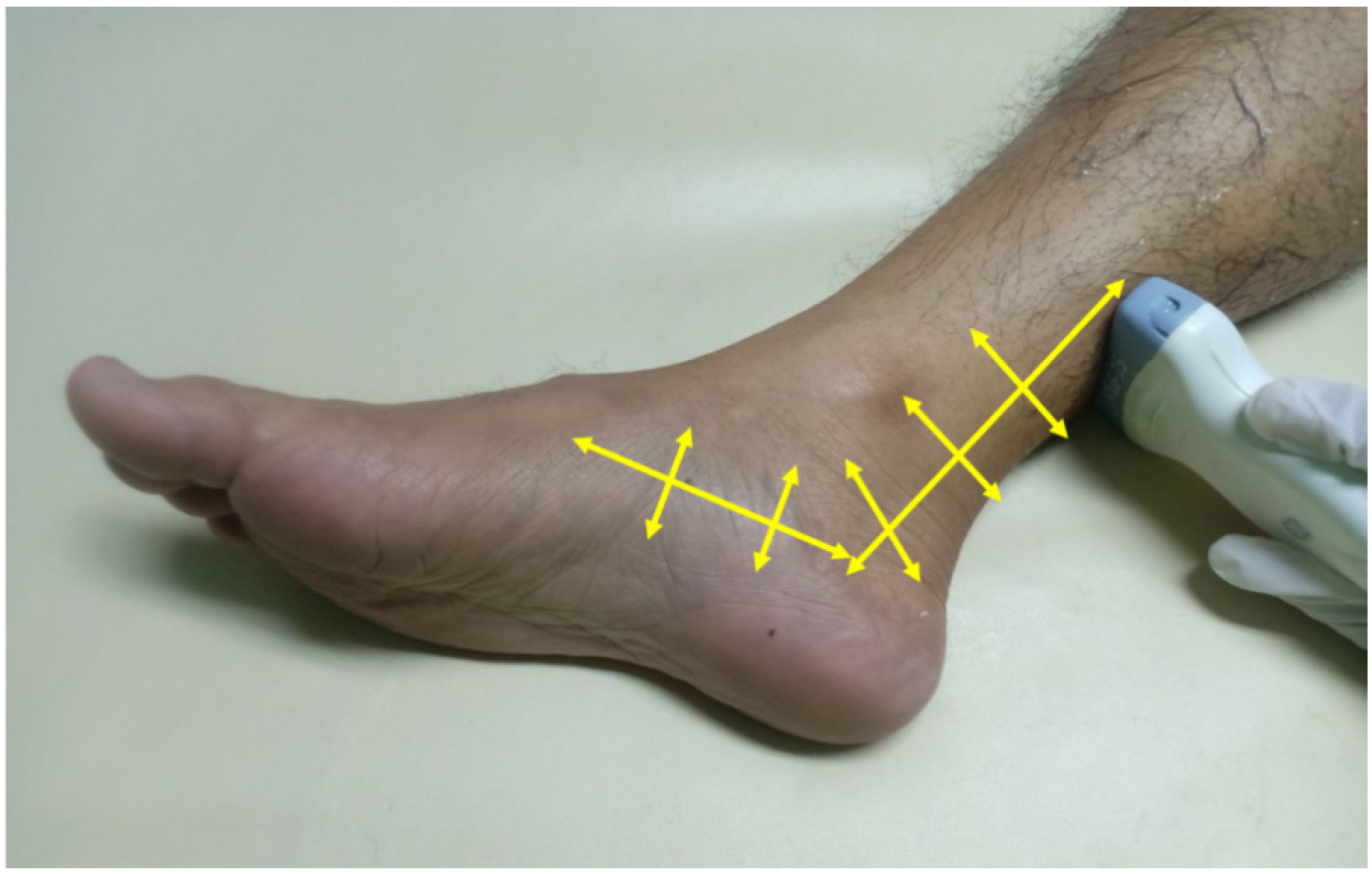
Transducer positioning for posterior tibial tendon ultrasound scanning positioning of lateral x-ray weight bearing foot.
